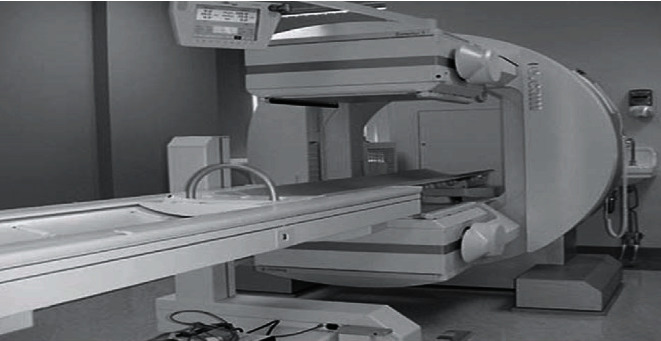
Dual-headed single-photon emission computed tomography (SPECT) system.
